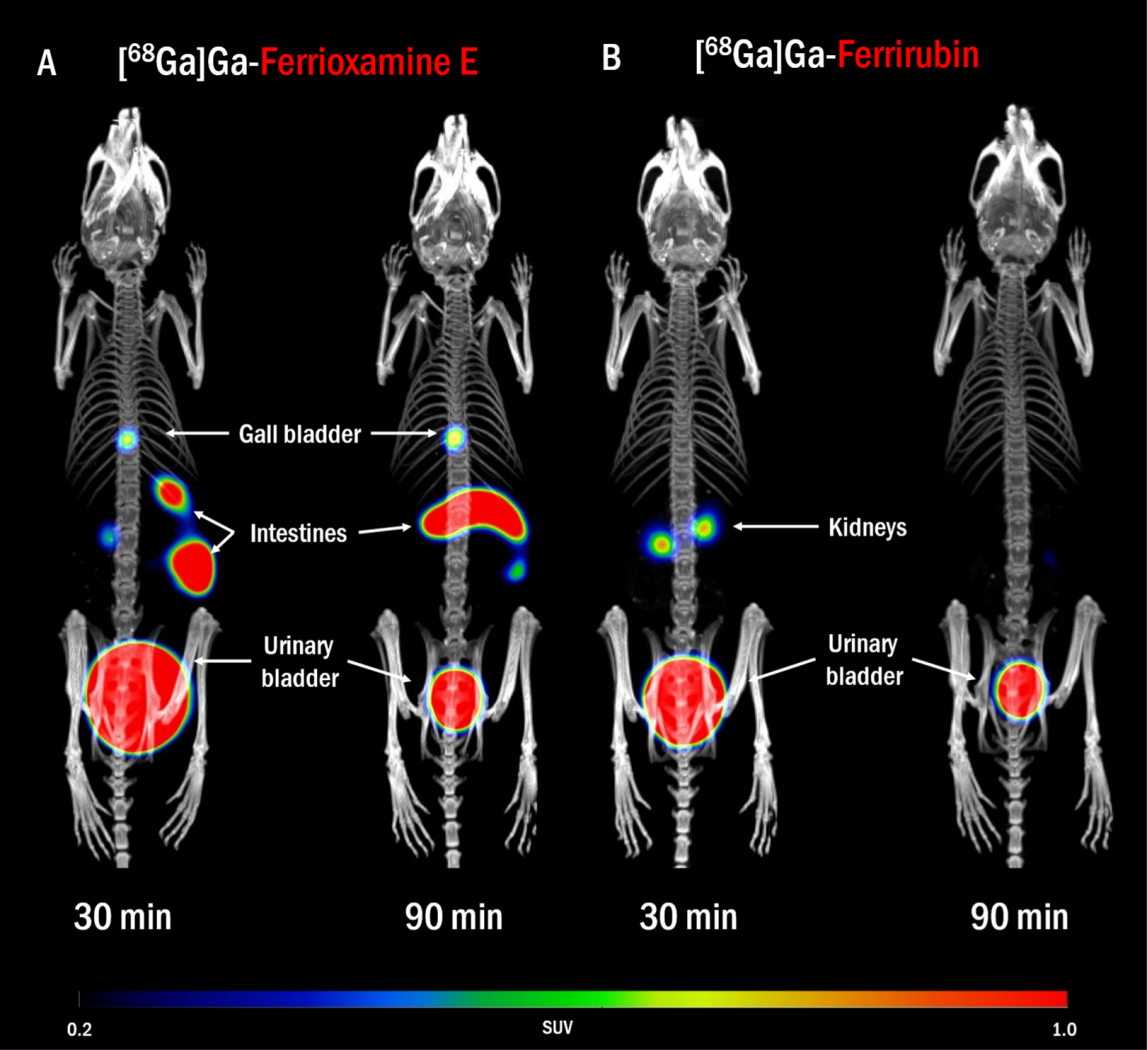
Maximum intensity projection (MIP) PET/CT
